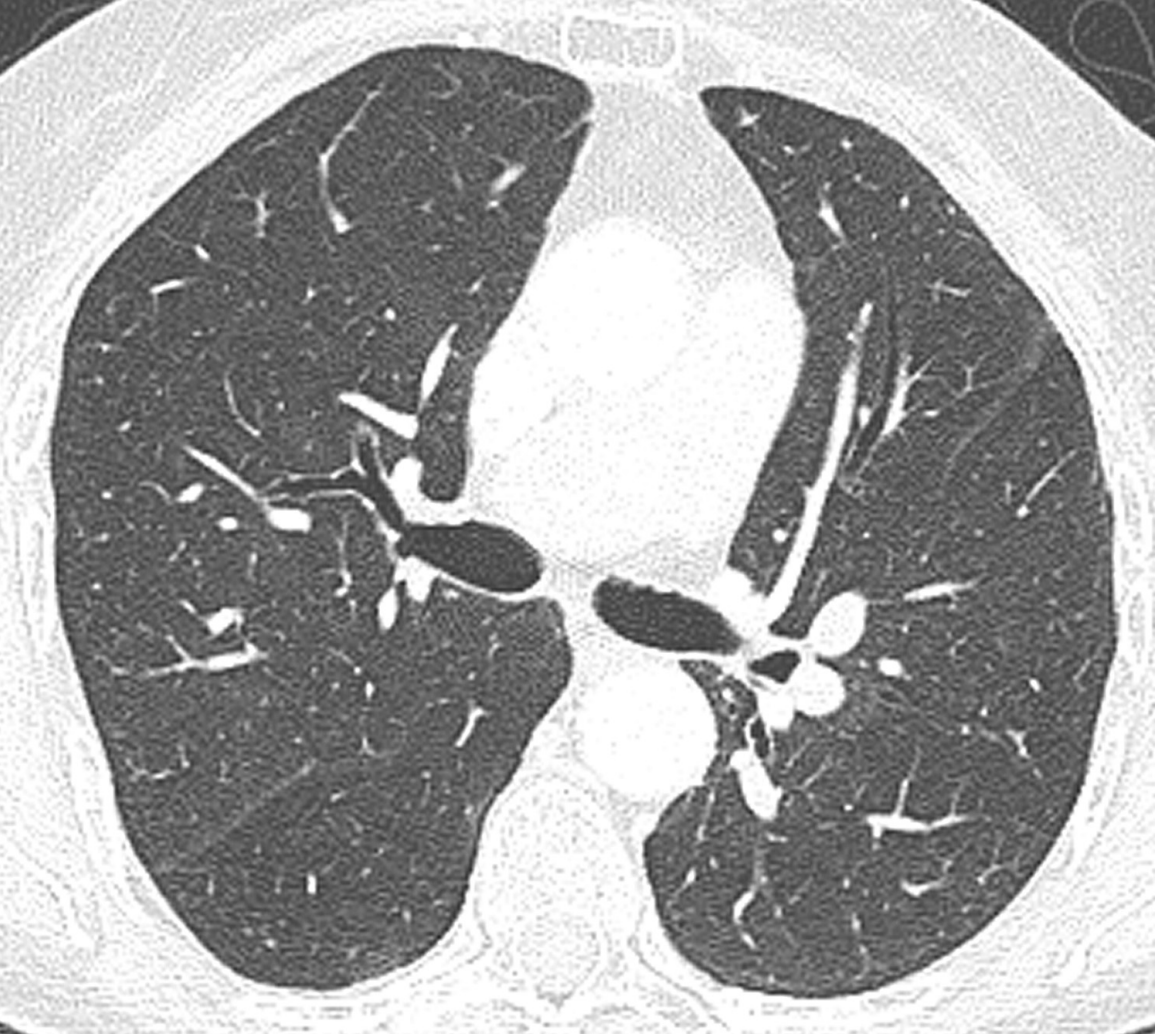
High-resolution transverse CT image at the same levels as figure 1 shows resolution of ground-glass opacity.
